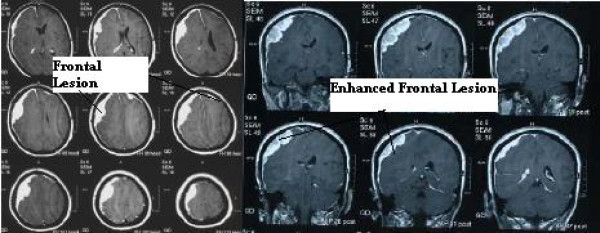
A brain MRI of the patient performed during her second admission showed an extradural lesion in the right frontoparietal region and a smaller lesion in the left frontal region (left) which became enhanced after a contrast injection (right).
